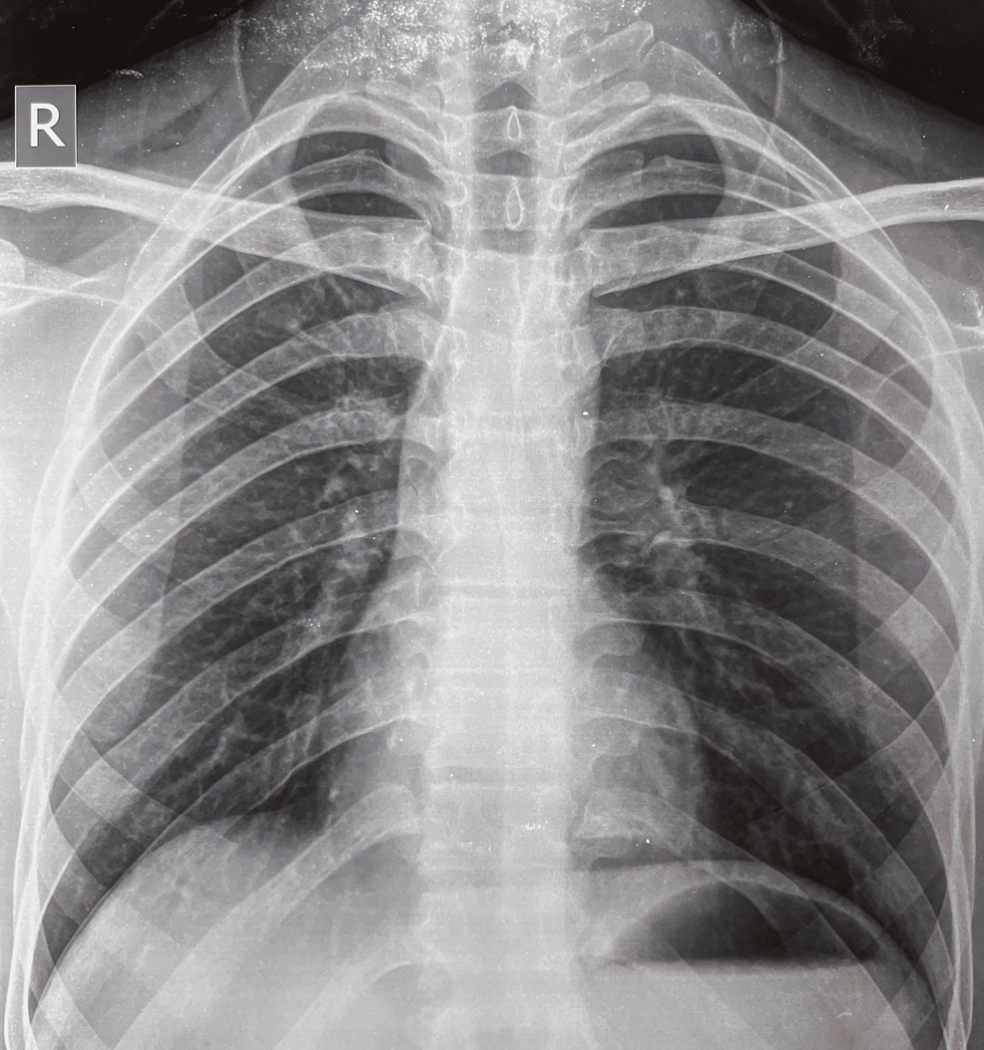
Chest X-ray of the patient showing clear lung fields.
